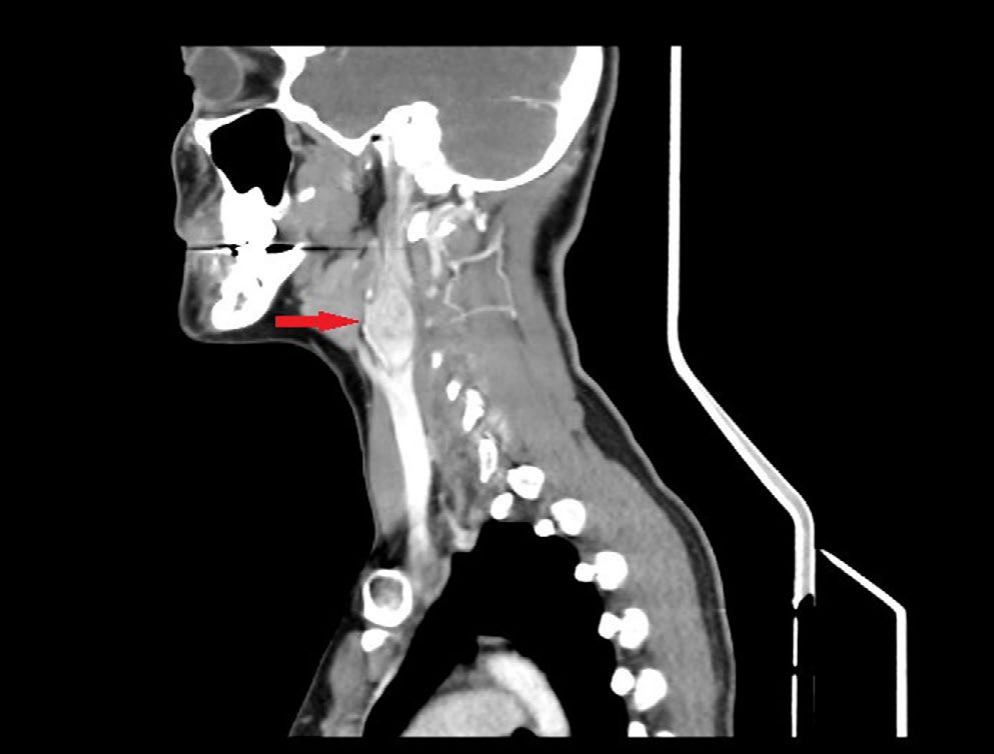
Head/neck CT demonstrating bilateral masses in the carotid bifurcations consistent with carotid body tumors
